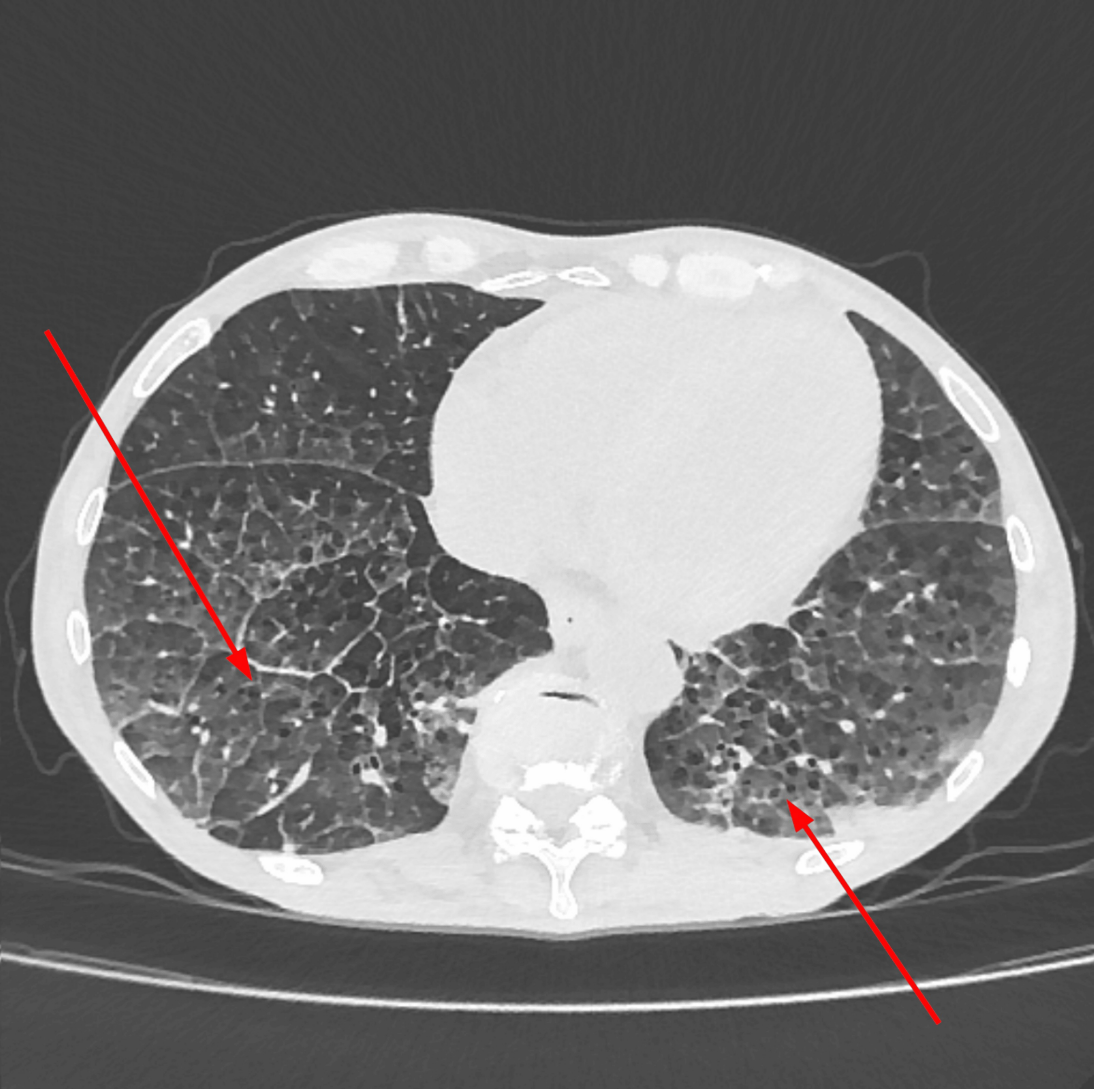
CT scan of the lungs. Crazy-paving patterns are indicated by red arrows.
